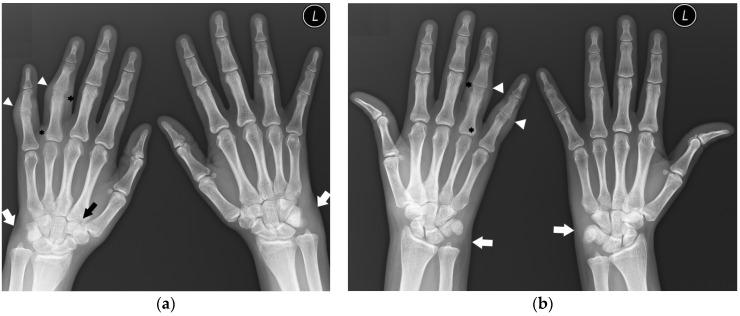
A 31-year-old female with PsA. PA (a) and Nørgaard radiographs (b) of bilateral hands. Note the sparring of the DIP joints. There is soft tissue swelling and contracture at the level of the fourth and fifth digits on the PIP joints of the right hand (arrowheads). Note the soft tissue swelling around both wrists, especially on the ulnar side (white arrows). There are erosions at the base and head of the right fourth digit proximal phalanx with discrete proliferative bone changes (stars). Note the narrowing of the left fourth PIP and right second CMC joint spaces with bone cysts at the base of the right second MC bone (black arrow).
